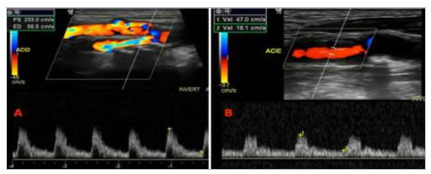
Duplex ultrasound of carotid stenosis. A: right internal carotid artery with stenosis greater than 70%. B: left internal carotid artery with stenosis less than 50% (criteria from Grant et al 2003
